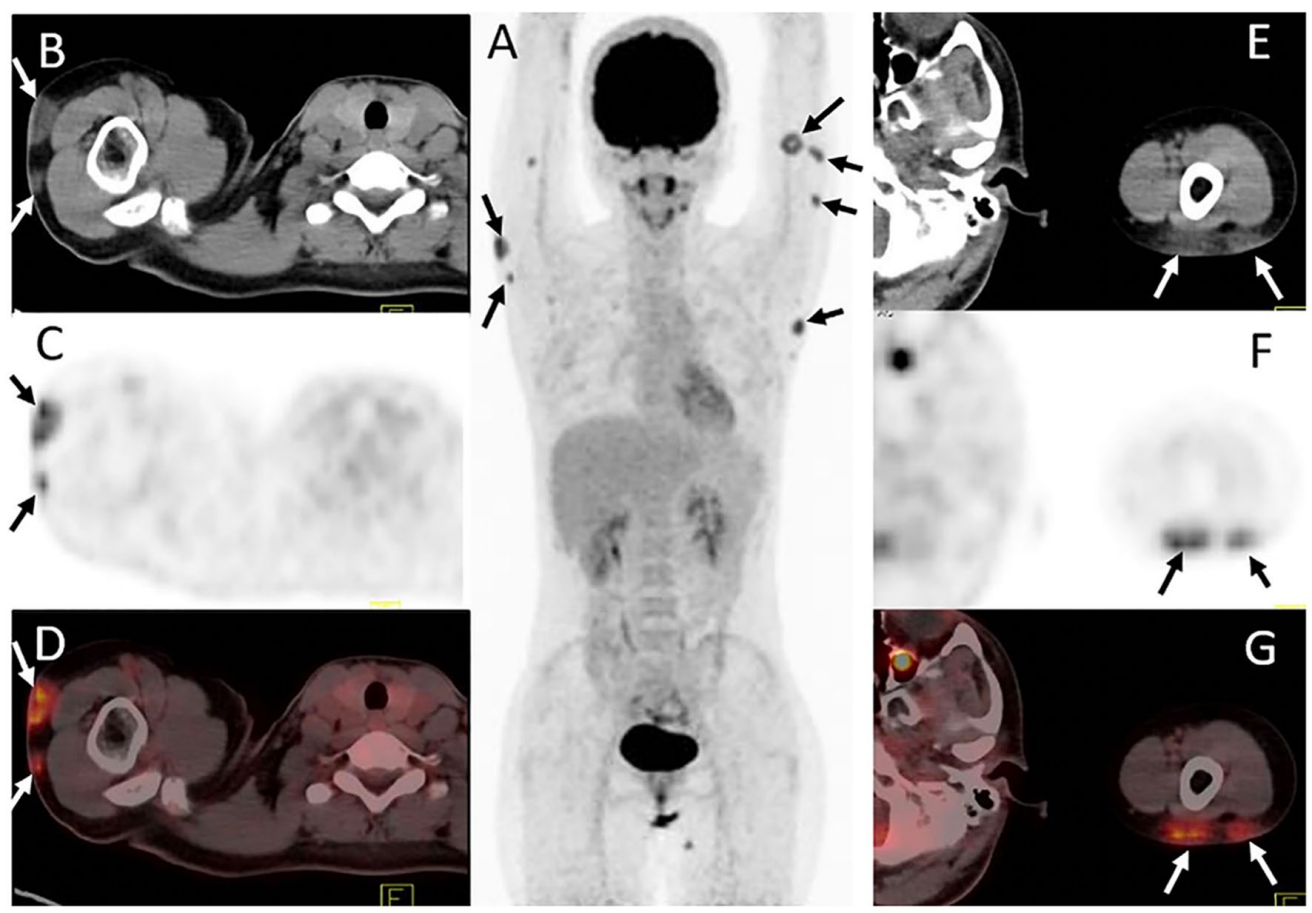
Subcutaneous SPTCL lesions are easy to miss on CT and visualization is significantly improved with ^18^F-FDG PET/CT. A 24-year-old female with subcutaneous panniculitis-like *T*-cell lymphoma located in the upper arms (black arrows). ^18^F-FDG PET/CT 3-dimensional maximum intensity projection image (A), axial CT (B, E) and axial fusion PET/CT (D, G) images reveal multiple subcutaneous lesions displaying high ^18^F-FDG uptake in the skin. Images courtesy of Jiang 2021 [6]
